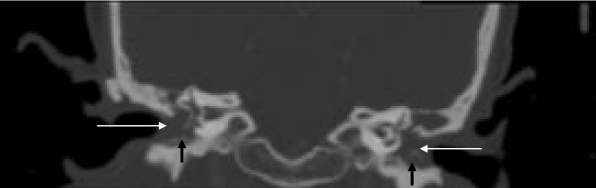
CT scan of temporal bone - Coronal reconstruction image showing soft tissue filling up the external auditory canal and the middle ear (long white arrows). There are also bony fragments seen (short black arrows).
